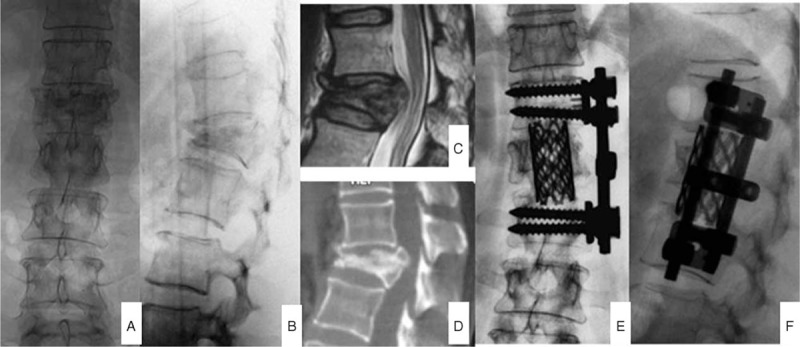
Female patient, 57-year old, was conducted anterior pathologic vertebra resection and internal fixation of titanium mesh supporting grafting bone after 1 month of lower back pain after trauma. Figures A and B represented the anteroposterior and lateral x-ray before operation, showing L1 fracture; figures C and D were MRI and CT before operation, demonstrating L1 fracture and Kümmell's disease; and figures E and F were x-ray after the anterior approach, displaying the fine internal fixation position and satisfactory kyphosis correction.
